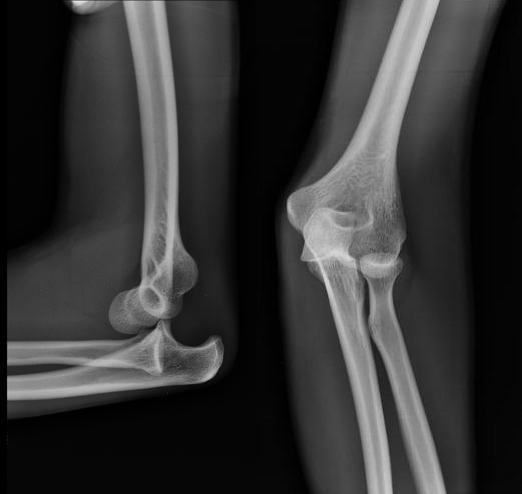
Radiographies de face et de profil montrant une luxation postéro-latérale du coude
